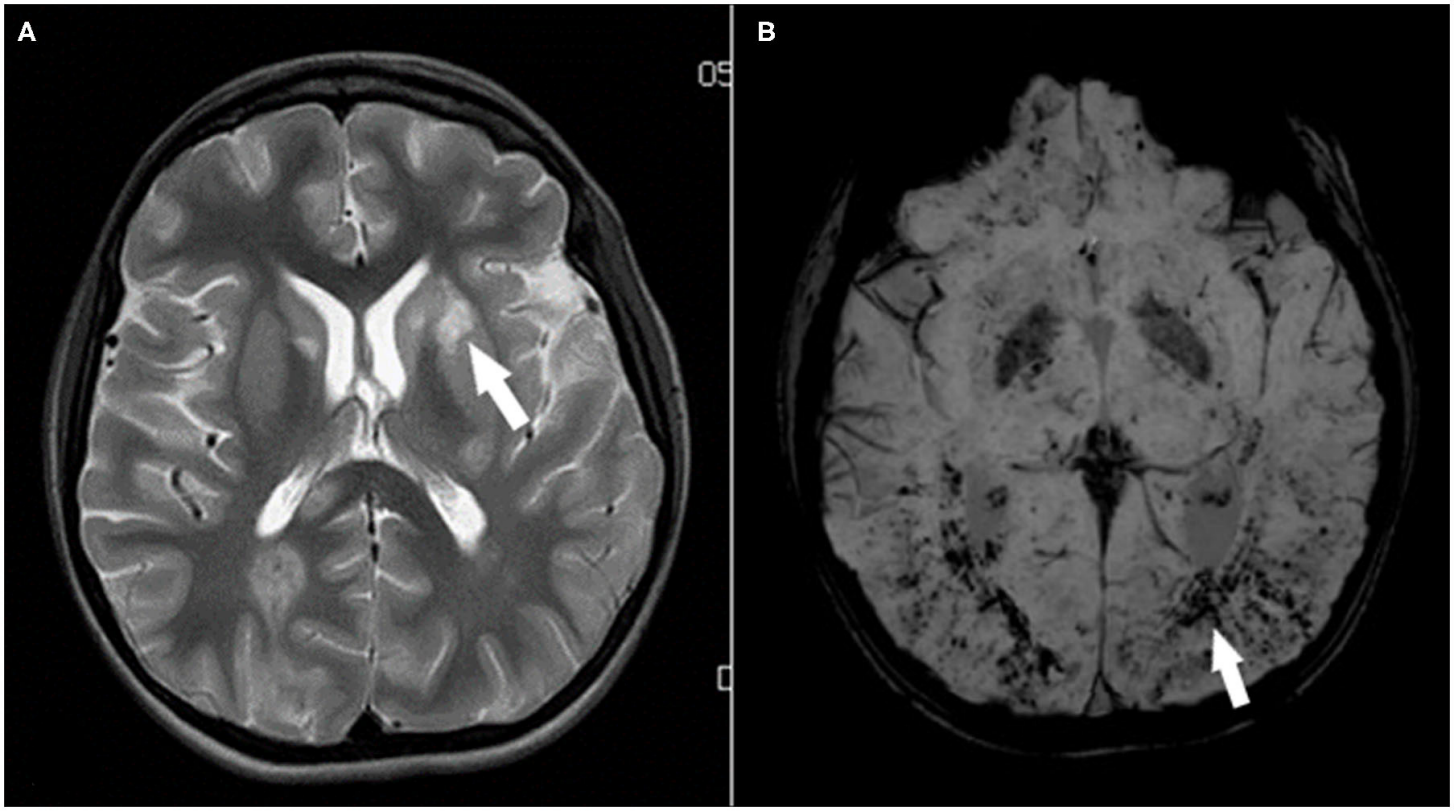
(A,B) Cerebral MRI 14 days after Lipiodol embolism. (A) Axial T2-weighted magnetic resonance imaging of the basal ganglia, showed diffuse high signal lesions, for example in the left putamen (arrow). Also, periventricular and subcortical white matter hyperintensities in both hemispheres, corresponding to edema after recent ischemia. (B) Susceptibility-weighted imaging (SWI) highlighted the presence of hemorrhagic components within the infarcted zones and diffuse, countless residual (micro-) hemorrhages (arrow) in supra- and infratentorial cerebral parenchyma.
